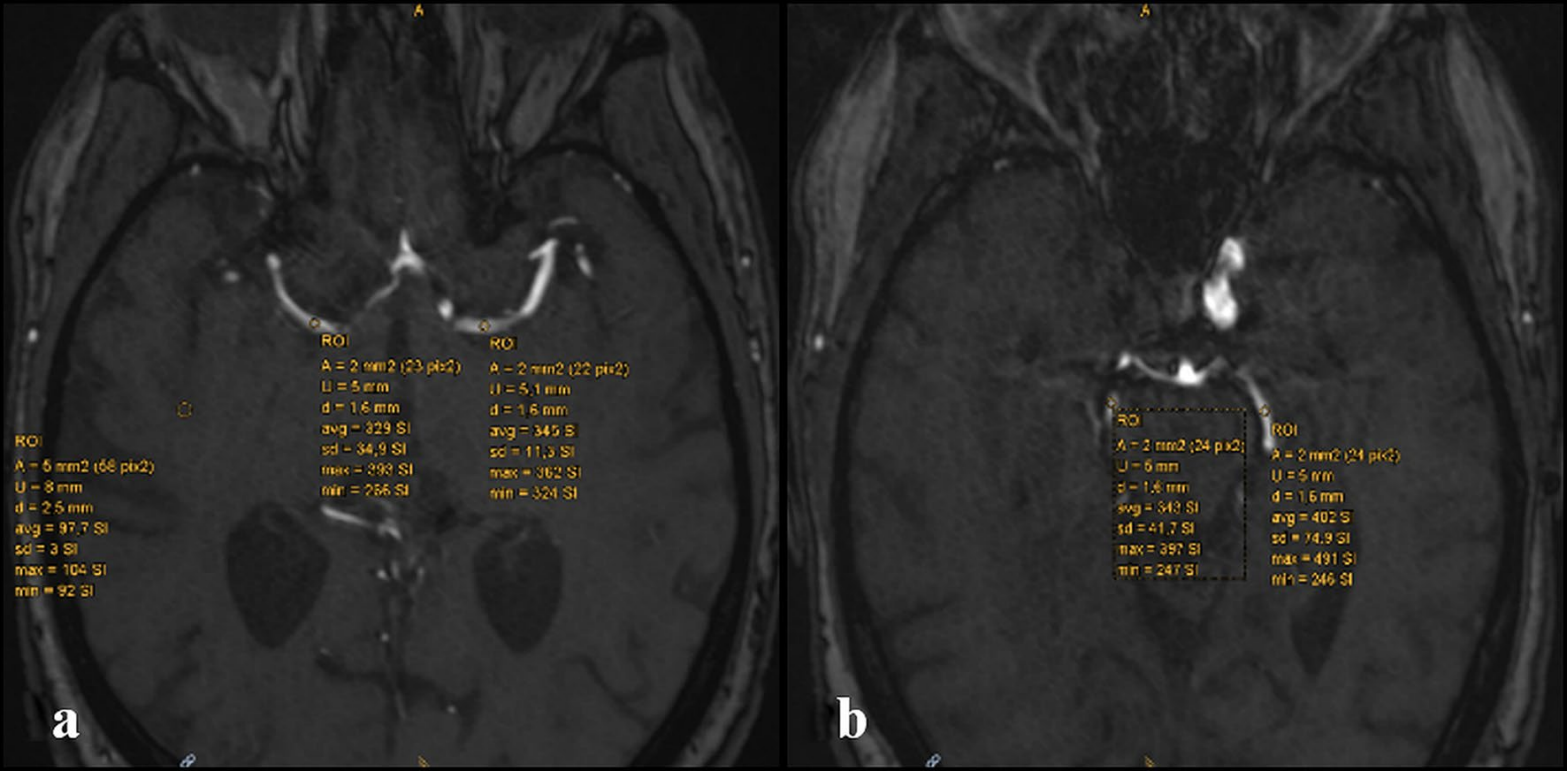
Starting from a 3D-MRA-TOF, a slice showing the bilateral MCA.M1 segments and another with bilateral PCA-P2-segments are chosen. For each artery, a ROI of 2mm^2^ is selected (2a-b). To adjust the measured values for variable background noise a reference signal intensity is measured in a 5mm2 ROI in avascular white matter (2a). 3D: three-dimensional; MRA: magnetic resonance imaging; TOF: time-of-flight; ROI: region of interest
